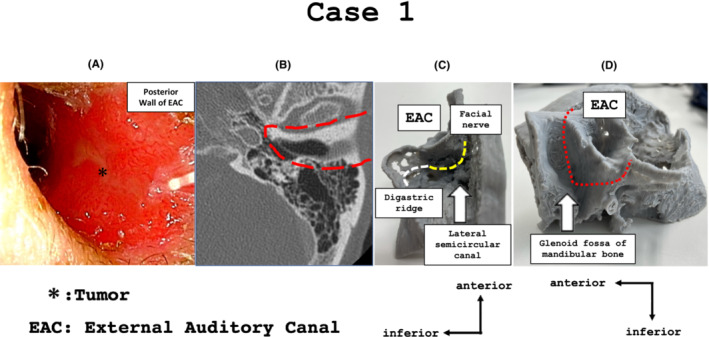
(A) An ulcerative lesion was observed from the posterior wall to the inferior wall of the left external auditory canal. (B) No obvious tumor was found on computed tomography (CT), and no bone destruction was observed. The dotted line is the resection line in CT axial scan. (C) We determined the depth of the antrum, lateral semicircular canals, middle cranial plate line, sigmoid sinus, digastric ridge, and facial nerve line. (D) The glenoid fossa of the mandible can be observed. Furthermore, the resection line can clearly be seen when we resect forward after performing mastoidectomy.
